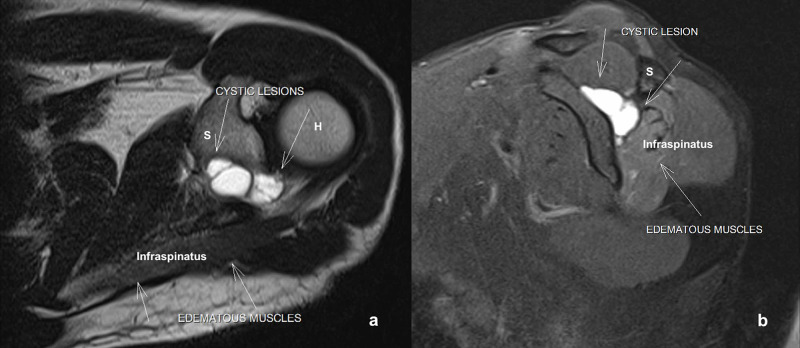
Magnetic resonance imaging. (a) T2 axial section showing multiple multiloculated spinoglenoid cyst (white arrow) in the left shoulder; H - humerus head; S - spine of scapula. (b) Sagittal section showing spinoglenoid cyst with the scapular spine (S) of the left shoulder and denervation of the infraspinatus muscle.
